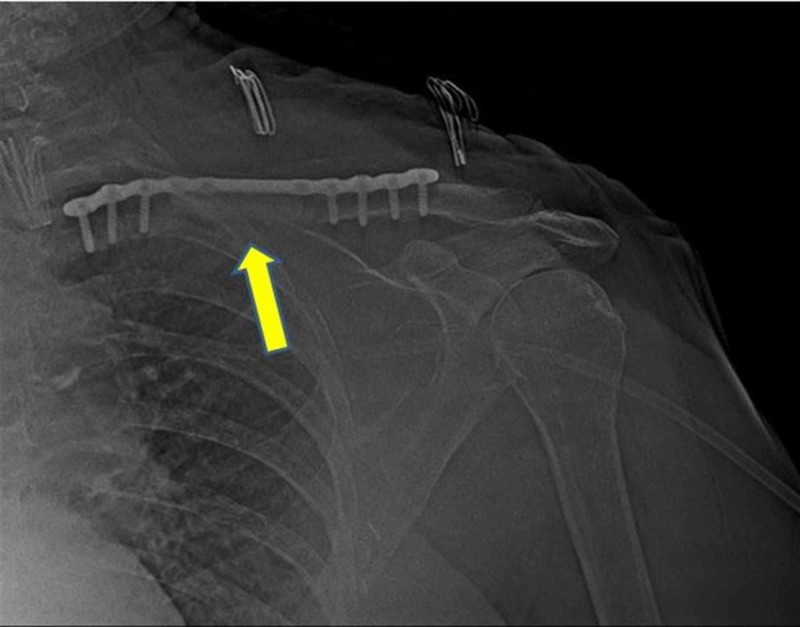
Postoperative X-ray of the left clavicle
